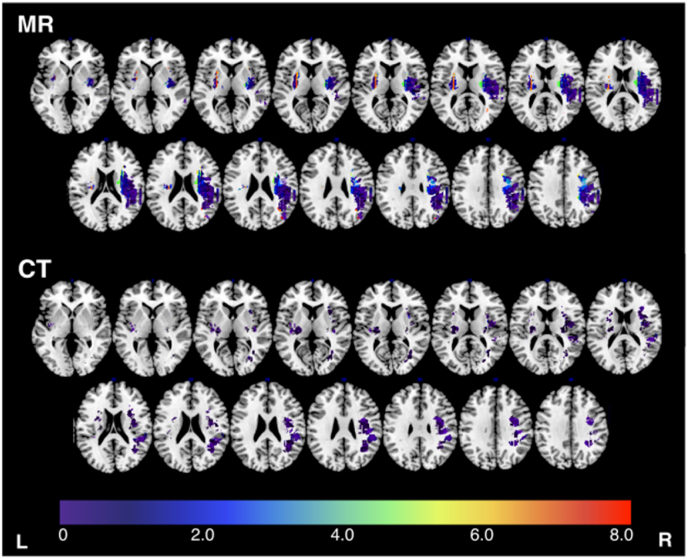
Target distance for CT and MR analyses.
